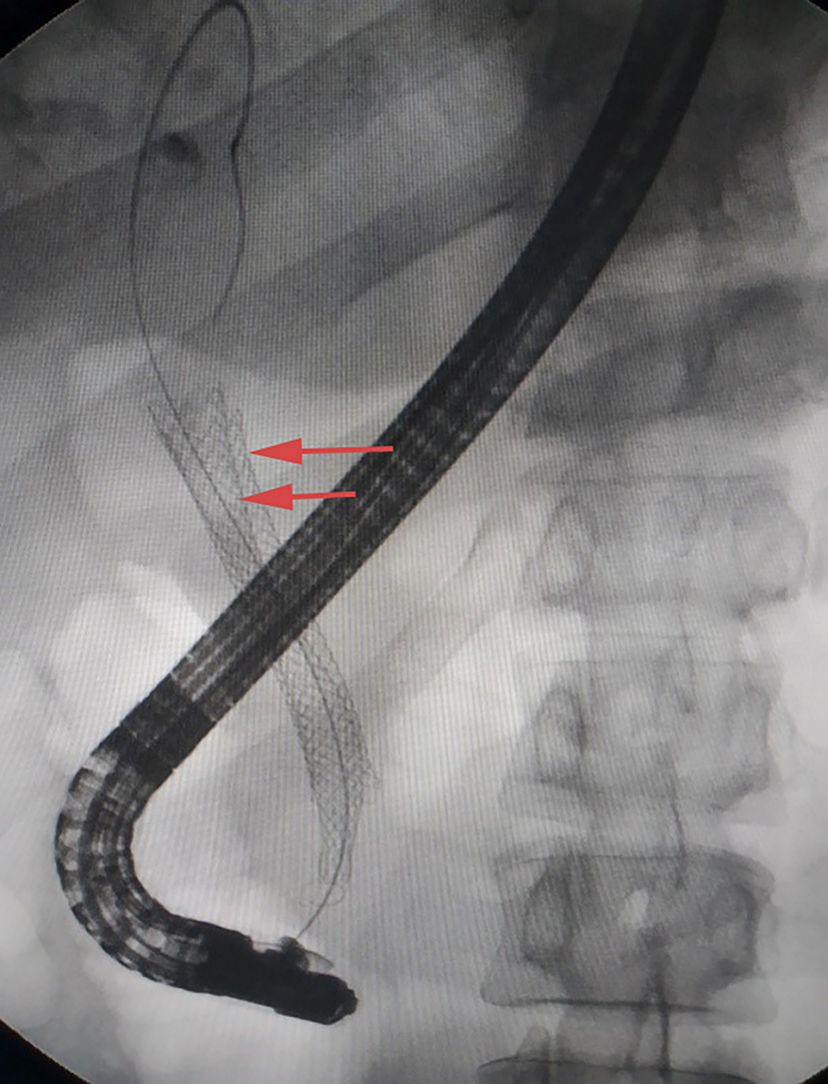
X-ray image showing double stent after insertion of the second biliary uncovered metal stent (yellow arrow).
